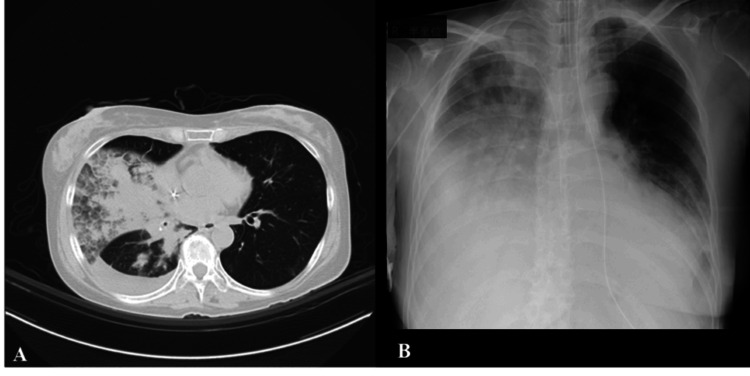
Imaging findings at intensive care unit admission. (A) CT findings. (B) Chest radiograph. Infiltrative shadows are observed throughout most of the right lung field CT: computed tomography
